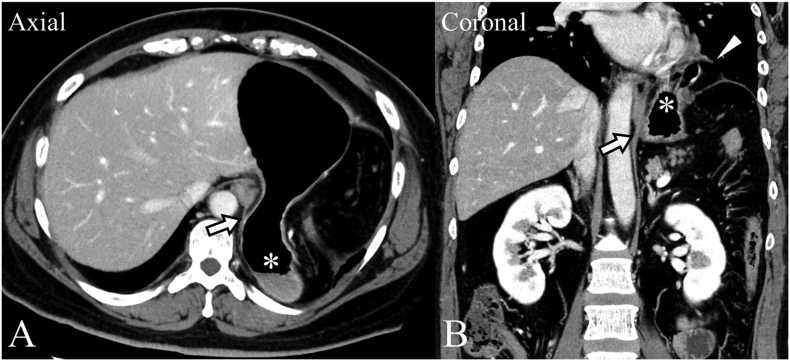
Preoperative contrast-enhanced computed tomography (CT). (A, B) Scan showing prolapse of the stomach into the thorax (*) from the outside of the left crus of the diaphragm (arrow). (B) A continuous membranous structure was observed from the diaphragmatic defect and covering the protruding part of the stomach (arrowhead).
